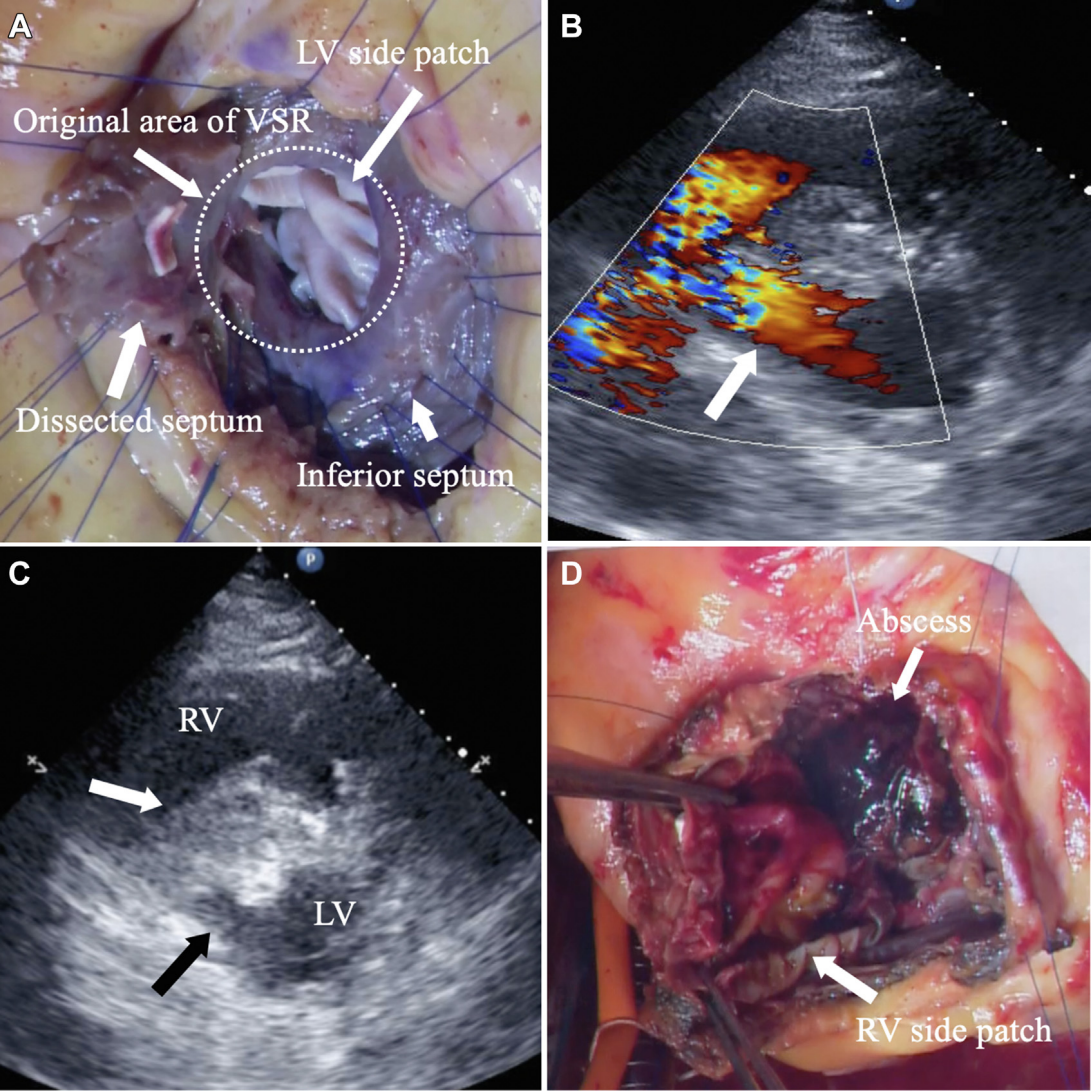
(A) Intraoperative photograph through right ventriculotomy at the initial operation shows the original area of ventricular septal rupture (VSR) and left ventricular (LV) side patch of double-patch repair. (B) Transthoracic color Doppler echocardiography shows relapse of ventricular septal rupture (arrow). (C) Transthoracic echocardiography shows large vegetation (white arrow) and collapse of the patch (black arrow). (D) Intraoperative image shows a large abscess (arrow) between the 2 patches. (RV, right ventricle.)
